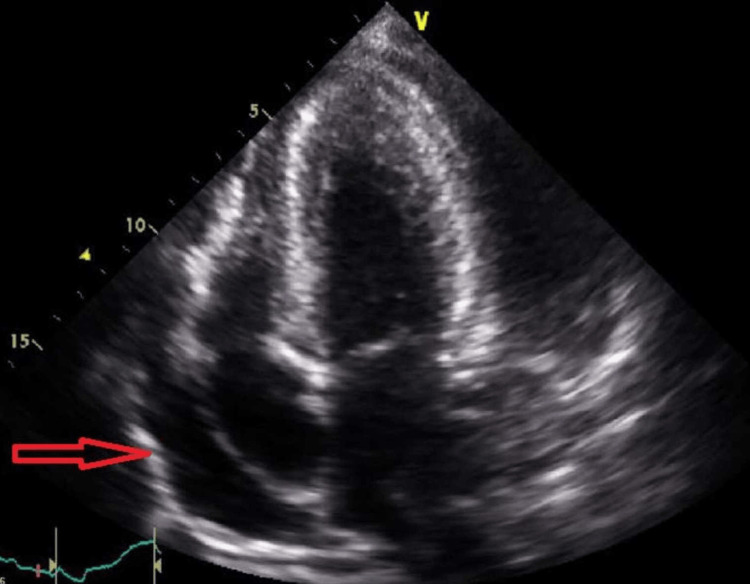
Echocardiography demonstrating (red arrow) a large pericardial effusion present on initial imaging.
